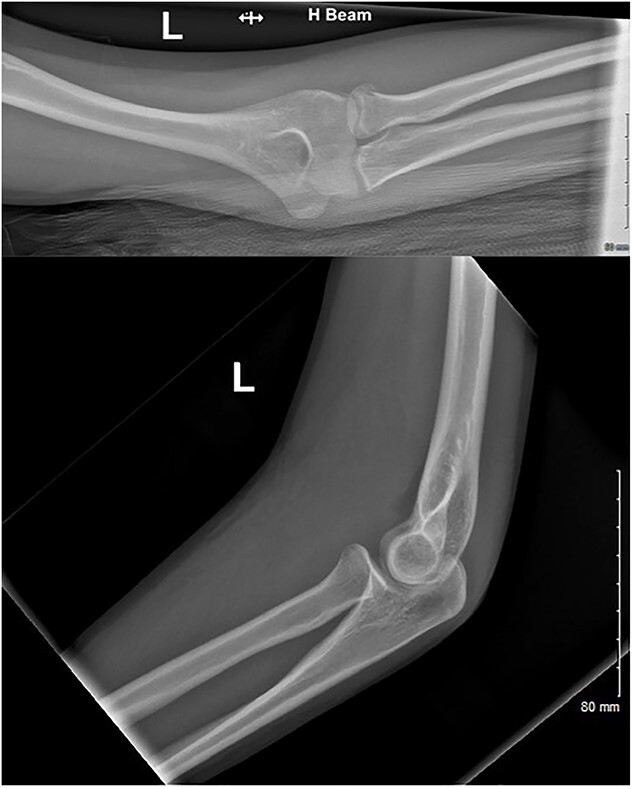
AP and lateral radiographs of the injury at first presentation to the emergency department.
